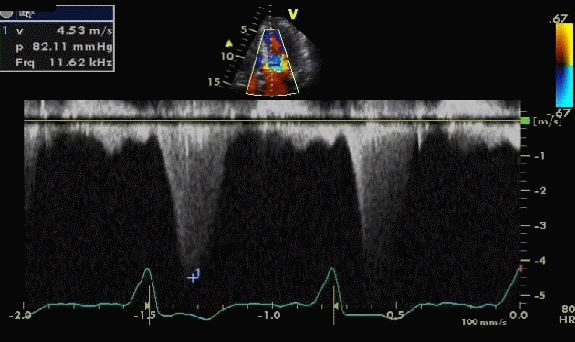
Continuous wave (CW) Doppler across the left ventricular outflow tract showing a gradient of 82.2 mmHg prior to insertion of pacemaker.
